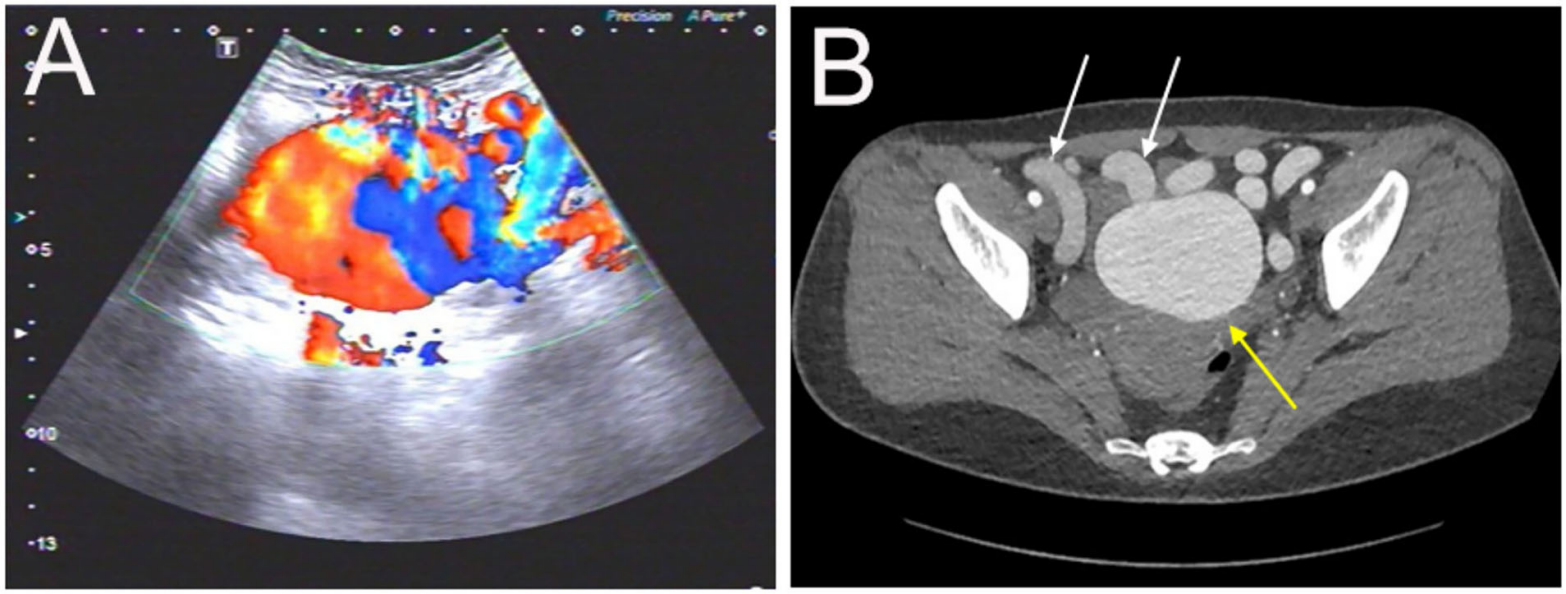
Evaluation of the abdominopelvic arteriovenous malformation (AVM) with color Doppler ultrasound and CT angiography. (A) The ultrasound revealed a pelvic mass with an abundant blood flow signal. (B) The axial view of CT angiography showed the nidus of AVM (yellow arrow) and dilated outflow veins (white arrows) (the aneurysm was measured as 72 ×56 mm).
